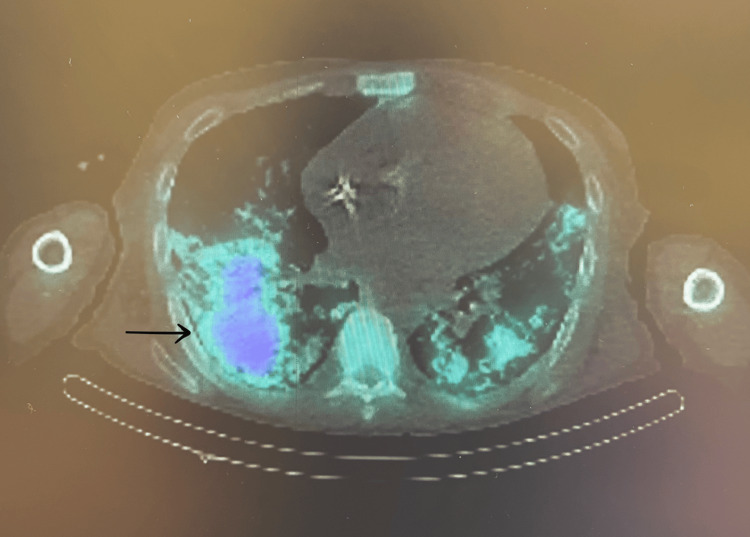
Bone scintigraphy with SPECT-CT showing increased tracer uptake in bilateral lung fields This finding, corresponding to ground-glass opacities on CT, is consistent with metastatic pulmonary calcification.
